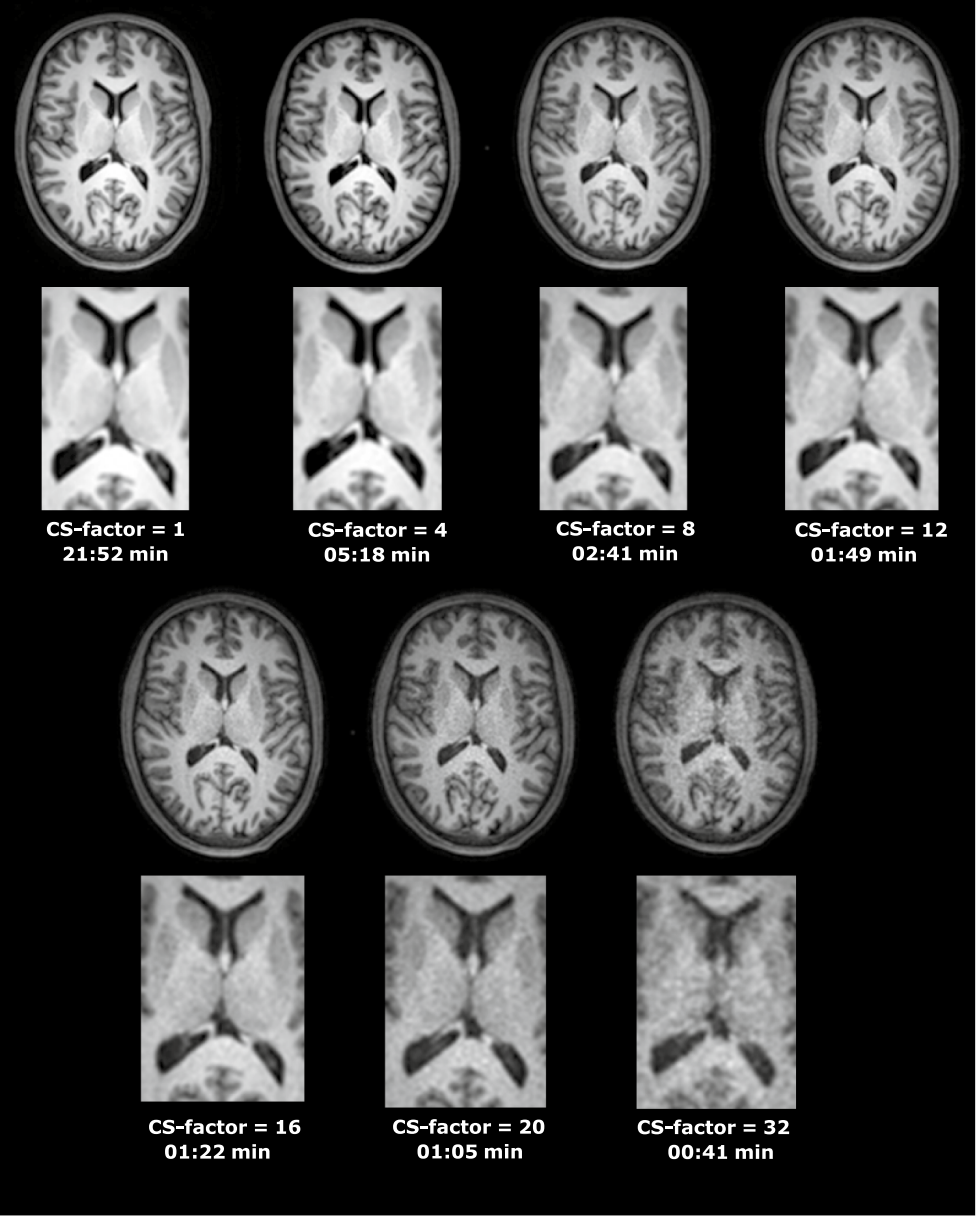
Sample axial reconstructions of the acquired 3D-T1-weighted brain images at increasing CS-factors of one of the subjects (enlarged view of the image center below each full FOV image). *CS* compressed SENSE
